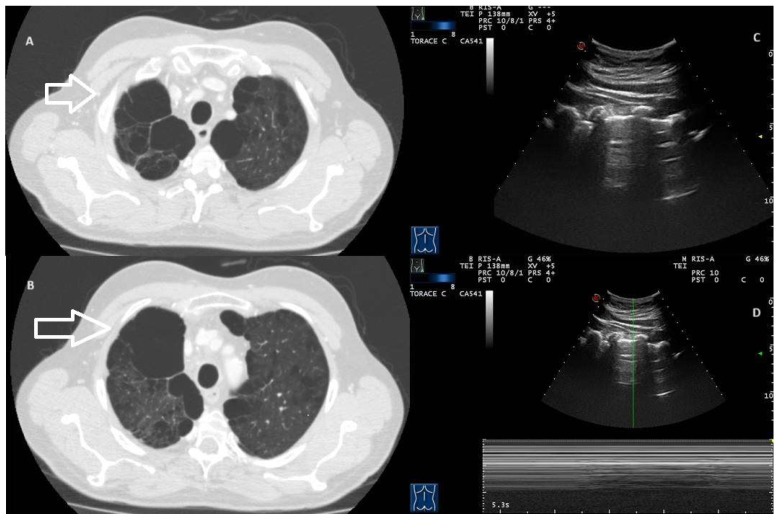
(A,B) Axial CT images showing extensive paraseptal emphysema characterised by voluminous thin-walled subpleural air bubbles in the right hemithorax (arrow). (C) Transthoracic ultrasound scan with a convex probe (7 MHz) corresponding to the area of paraseptal emphysema visualised on CT. Videoclip 1 shows the absence of a gliding sign. (D) Transthoracic ultrasound scan in M-mode showing the “bar-code” sign (absence of movement).
